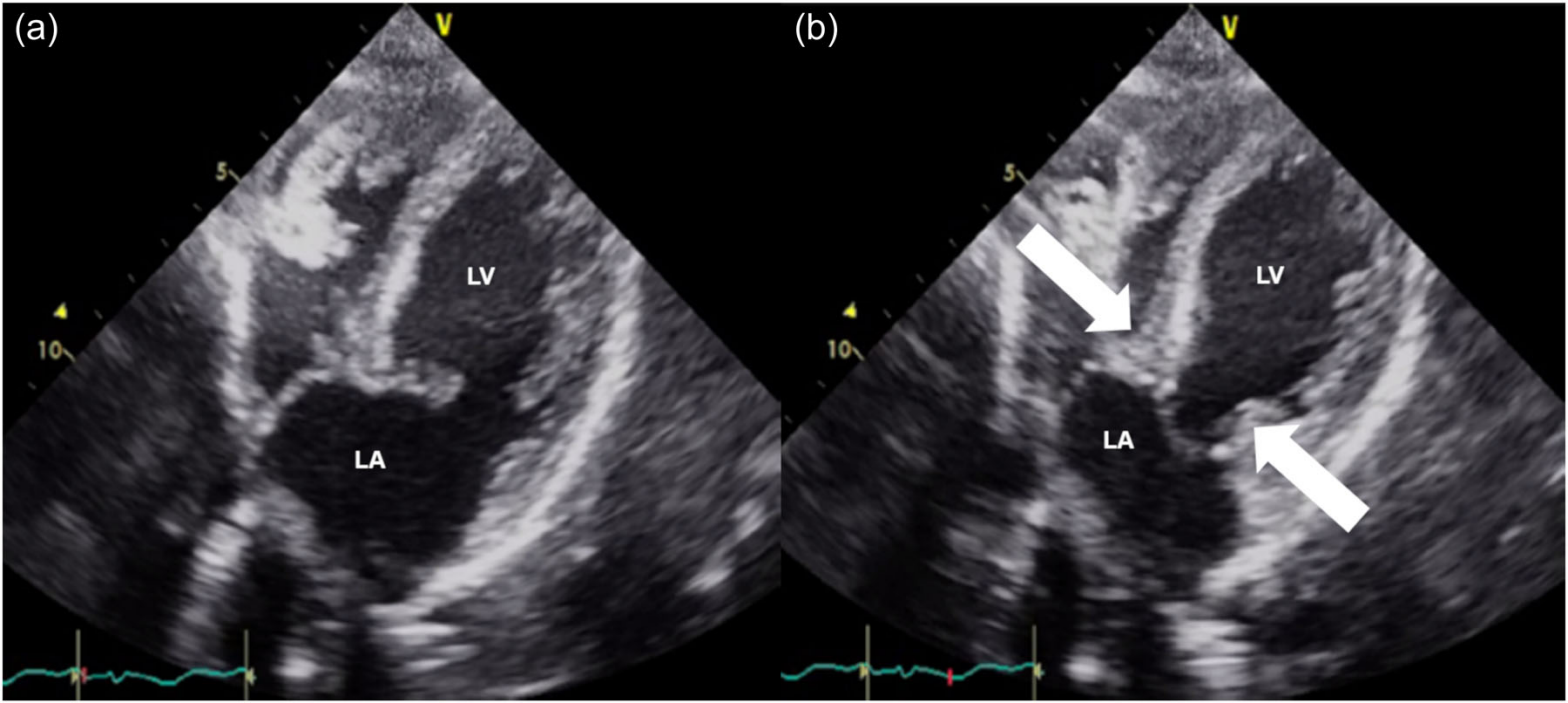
Echocardiography of TCM. Echocardiography shows akinesia around the apex and hypercontraction of the basal segments (arrows): (a) diastolic phase and (b) systolic phase. LA, left atrium; LV, left ventricle
